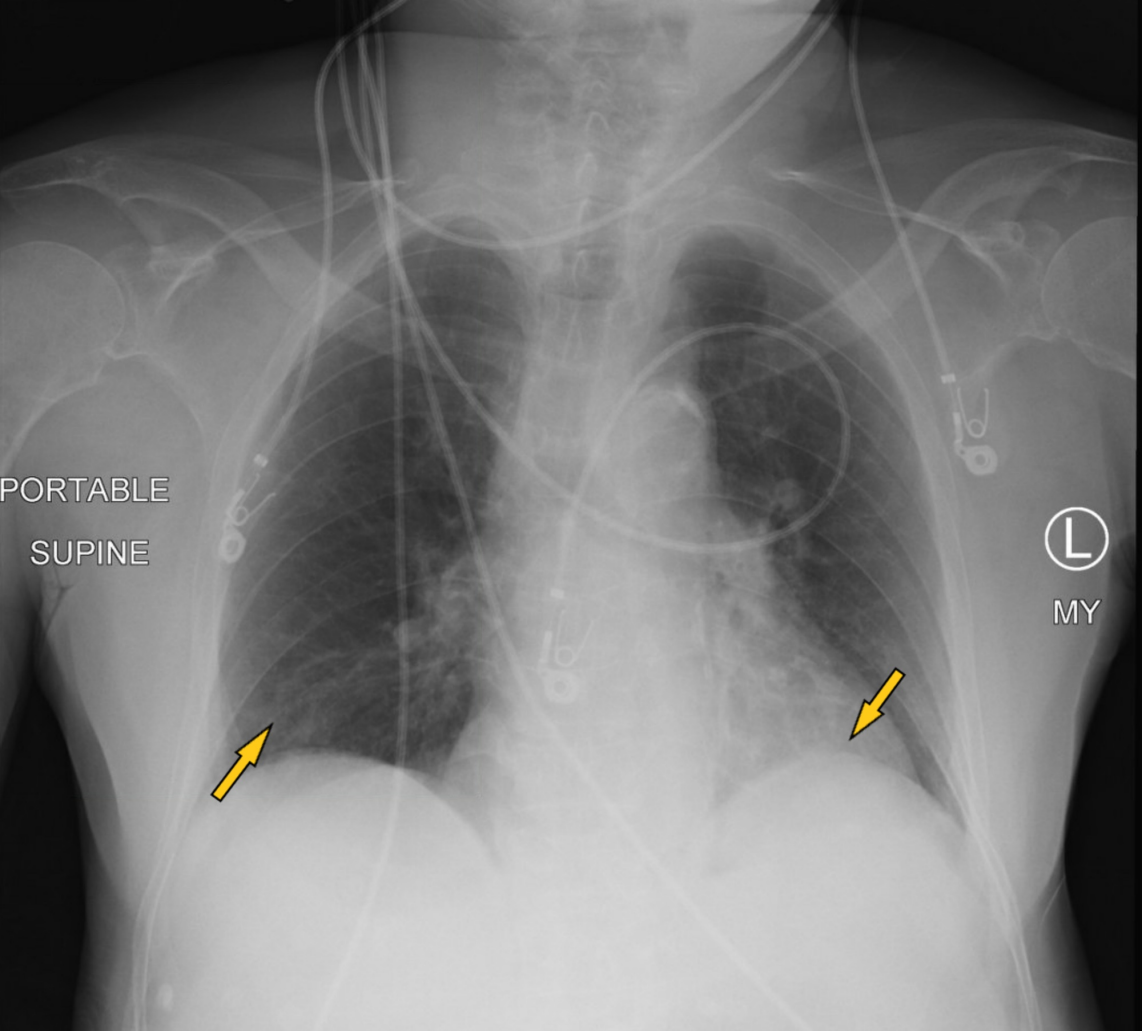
Chest X-ray showing bibasilar small airspace disease.
